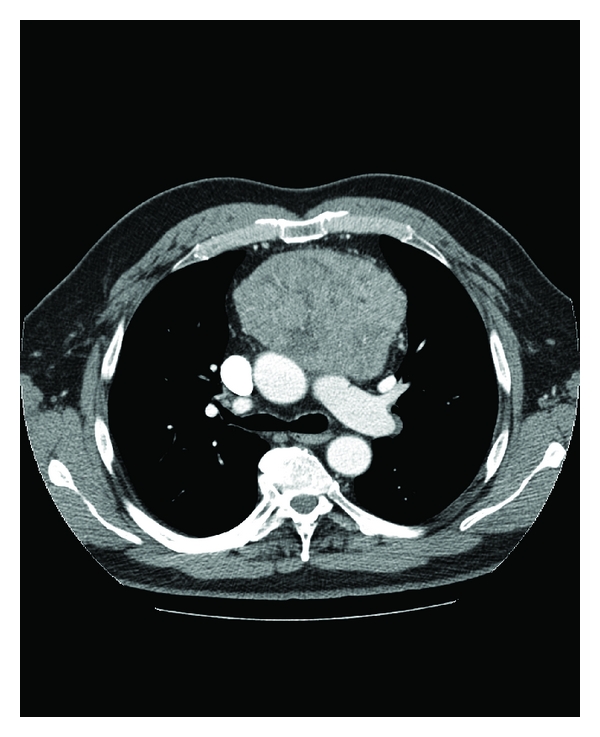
CT scan of the chest revealing a large heterogenous mass in the anterior mediastinum.
